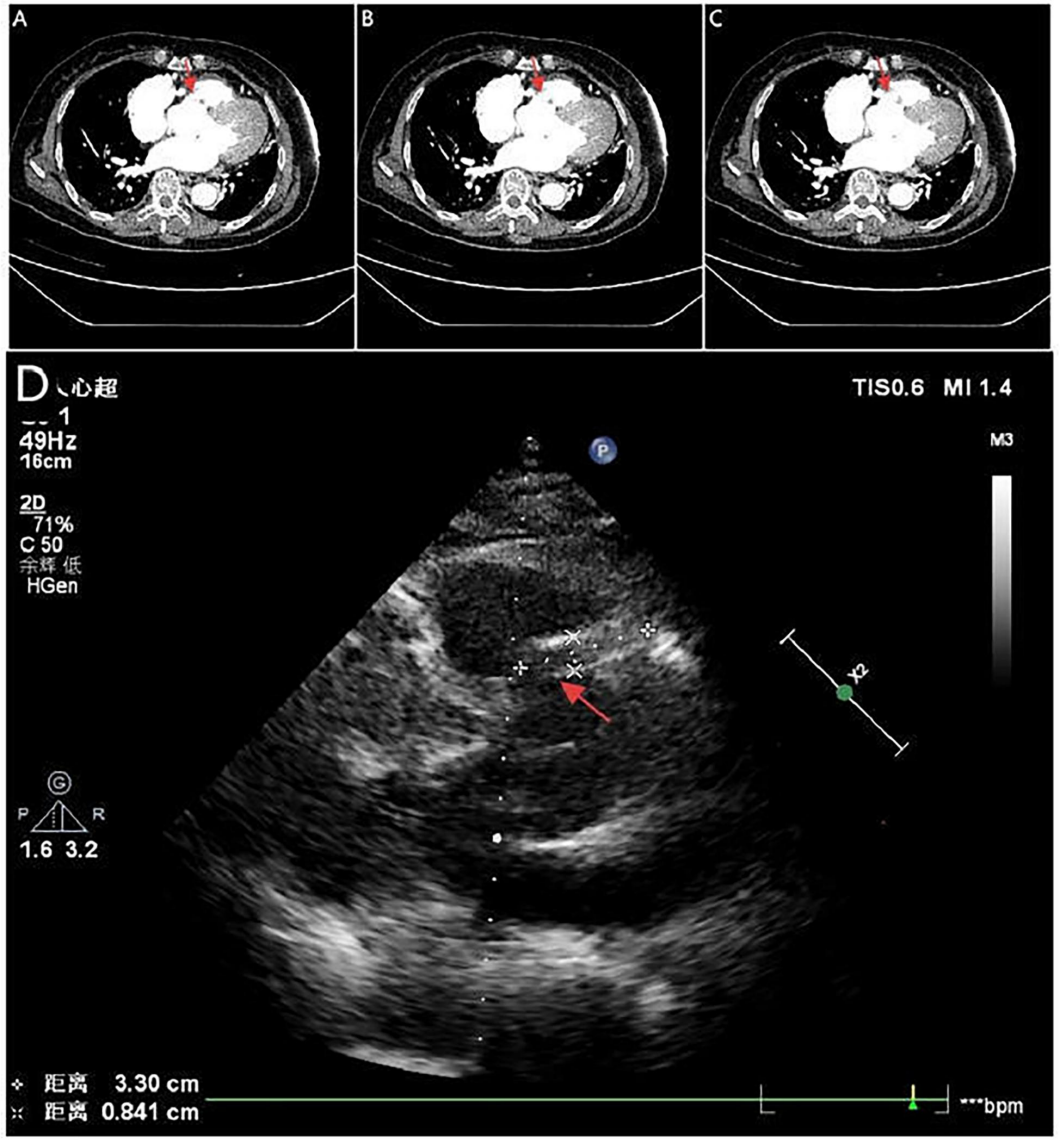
Serial CTA images (A–C) show located right coronary sinus of valsava dissection (red arrow). Echocardiography (D) demonstrates an intramural hematoma originating at the ostium of the RCA within the right coronary sinus, with partial extension into the proximal RCA (red arrow).
